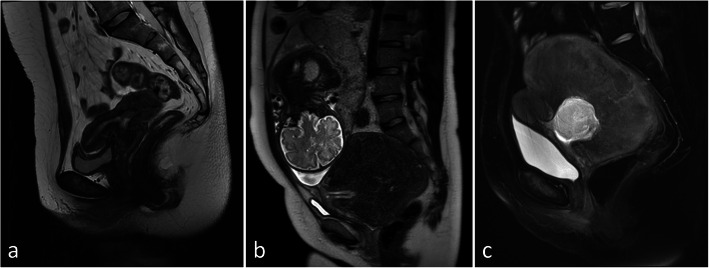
T2-weighted magnetic resonance imaging (MRI) of the uterus. MRI shows a myomatous mass with homogenous intensity from the normal uterine posterior wall close to the internal ostium of the uterus, which shaped the inner pouch structure (a). The myomatous mass increases at gestational week 32 (b). Post-cesarean section, the myomatous mass is as large as that observed during pregnancy, with an increase in the inner fluid (c)
